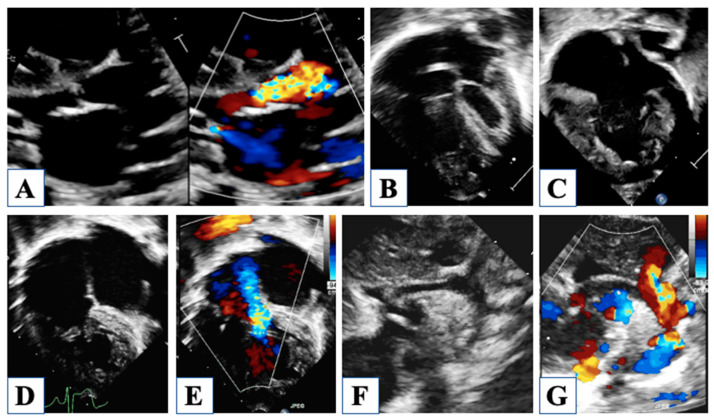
Severe aortic valve stenosis is shown in panel (A) from a parasternal long-axis view. Severe mitral valve hypoplasia with evident endocardial fibroelastosis of the left ventricle in panel (B). Four-chamber view in panel (C) shows a double inlet right ventricle with virtually absent left ventricle. Mitral atresia in panel (D,E) is associated with dysplasia of the tricuspid valve, with moderate regurgitation. Aortic valve atresia with severely hypoplastic ascending aorta is shown in panel (F). Panel (G) shows aortic arch hypoplasia with ductal dependency and reverse flow in the aortic arch.
